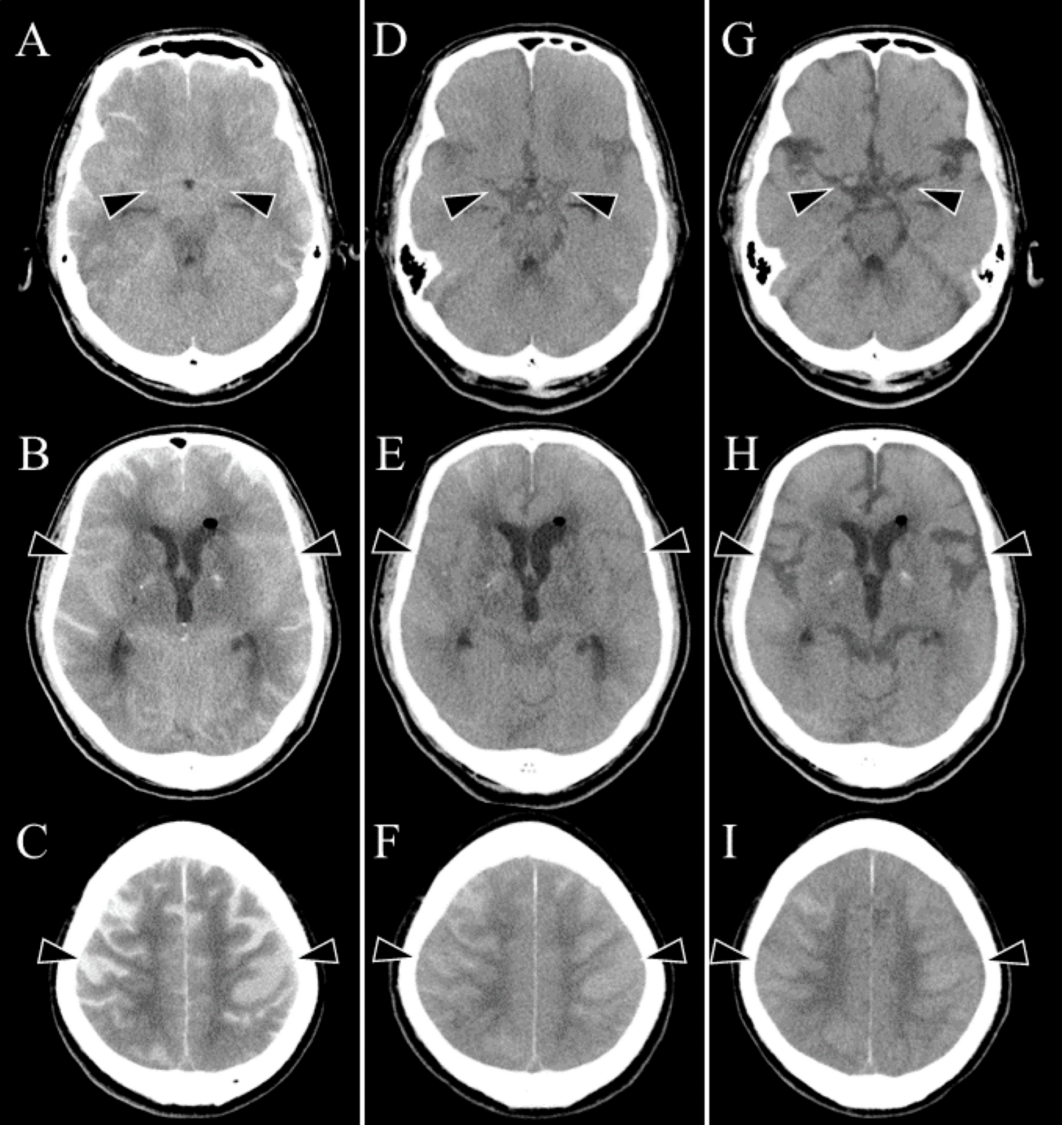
Serial non-contrast head CT demonstrating progressive decrease in subarachnoid hyperattenuation. (A–C) Initial CT obtained at the referring hospital (Day 0, approximately 32 hours after myelography) shows hyperdense areas within the subarachnoid spaces (arrowheads), mimicking subarachnoid hemorrhage. (D–F) Follow-up CT at our hospital (Day 1, approximately 40 hours after myelography) demonstrates a reduction in both the extent and attenuation of the hyperdense lesions (arrowheads). (G–I) Subsequent CT (Day 1, approximately 48 hours after myelography) shows further decrease in hyperattenuation (arrowheads).
